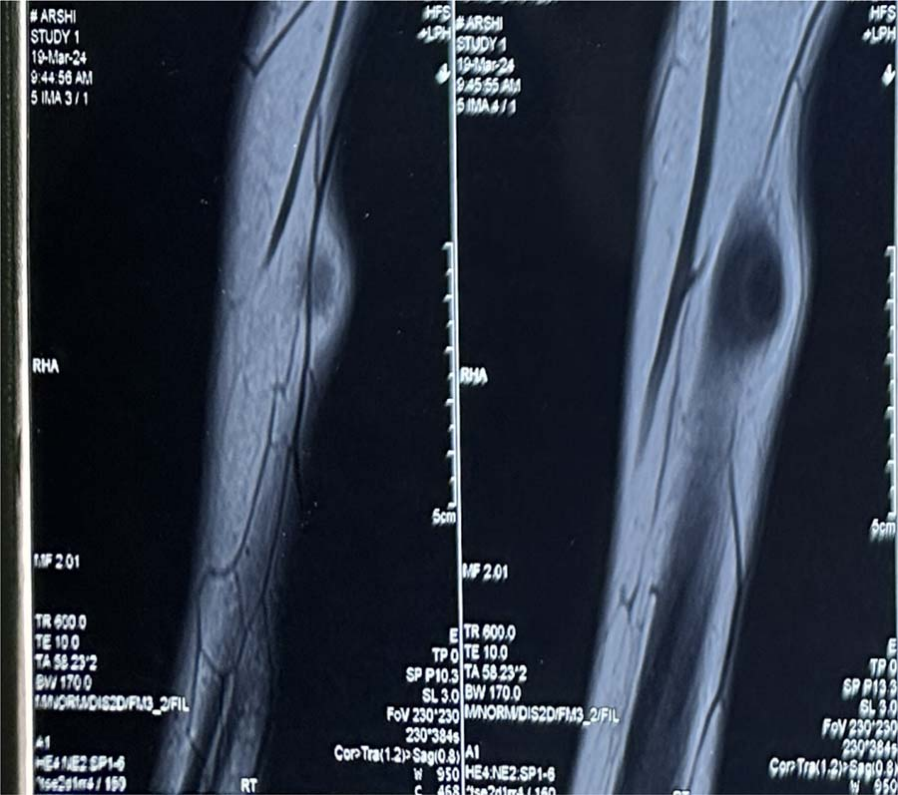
MRI Soft tissue of right forearm: suggestive of well-defined cystic lesion with eccentric mural nodule in intramuscular plane with mild perilesional oedema.
